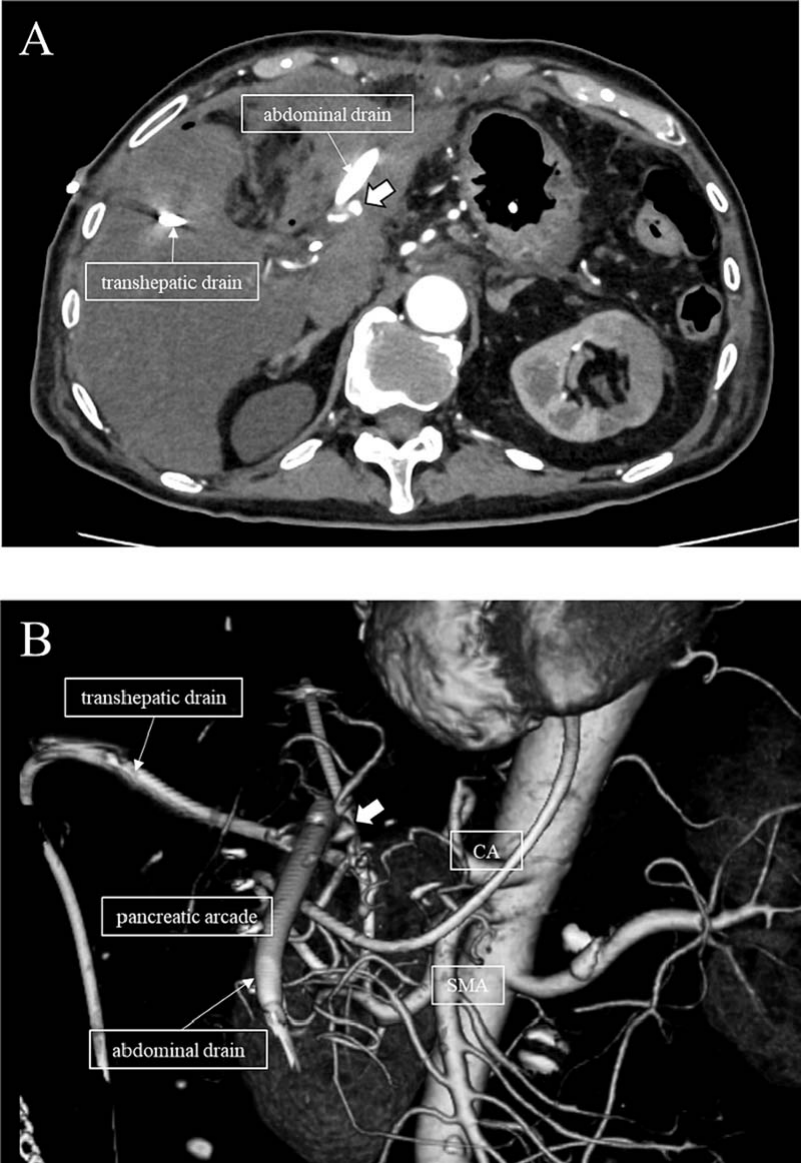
(A, B) CT on postoperative Day 56. Contrast-enhanced CT shows a pseudoaneurysm at junction of the GDA and PHA (arrow).
